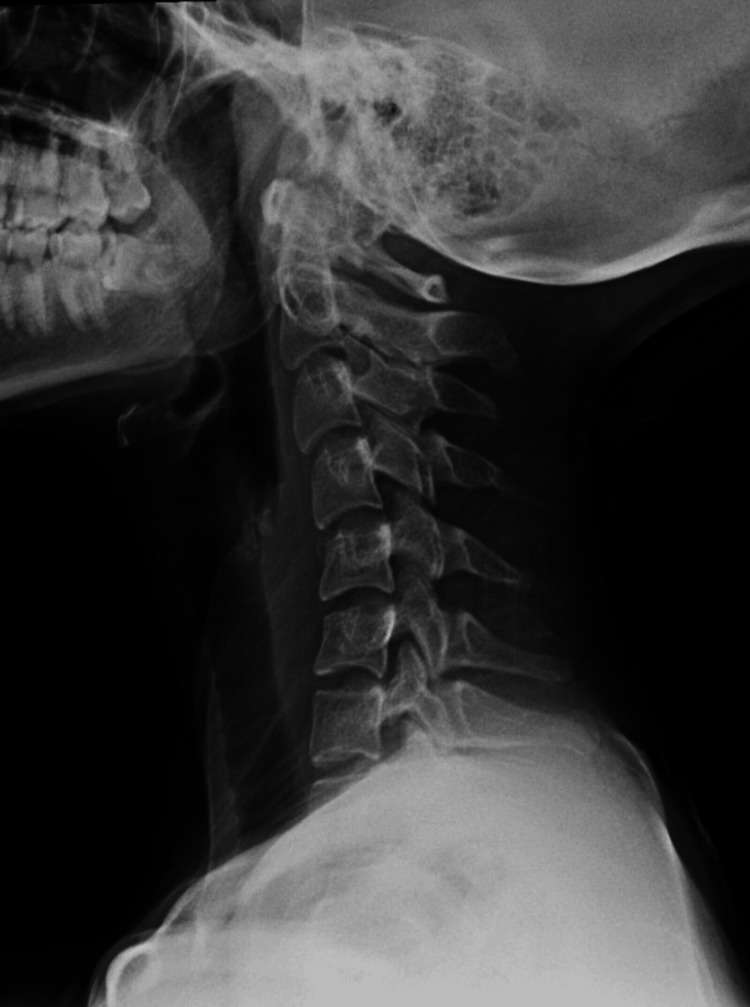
Neck X-ray shows no presence of soft tissue air or any fluid collection.
